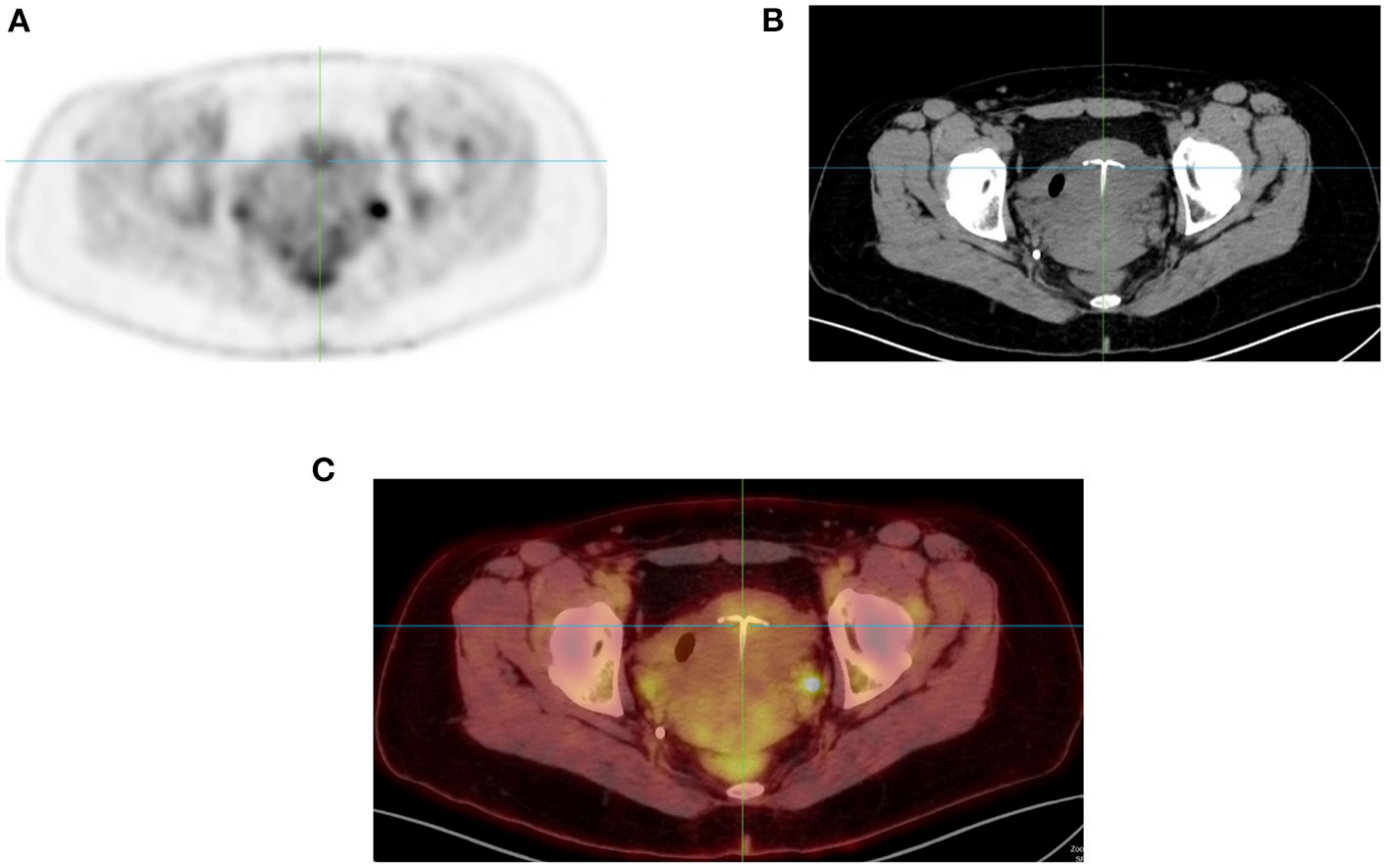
Uptake in intrauterine device (IUD). FDG PET MIP image in axial plane (A) showing focal uptake (Arrow) in anterior uterus. Corresponding CT (B) and combined PET/CT (C) confirms the uptake in the IUD.
